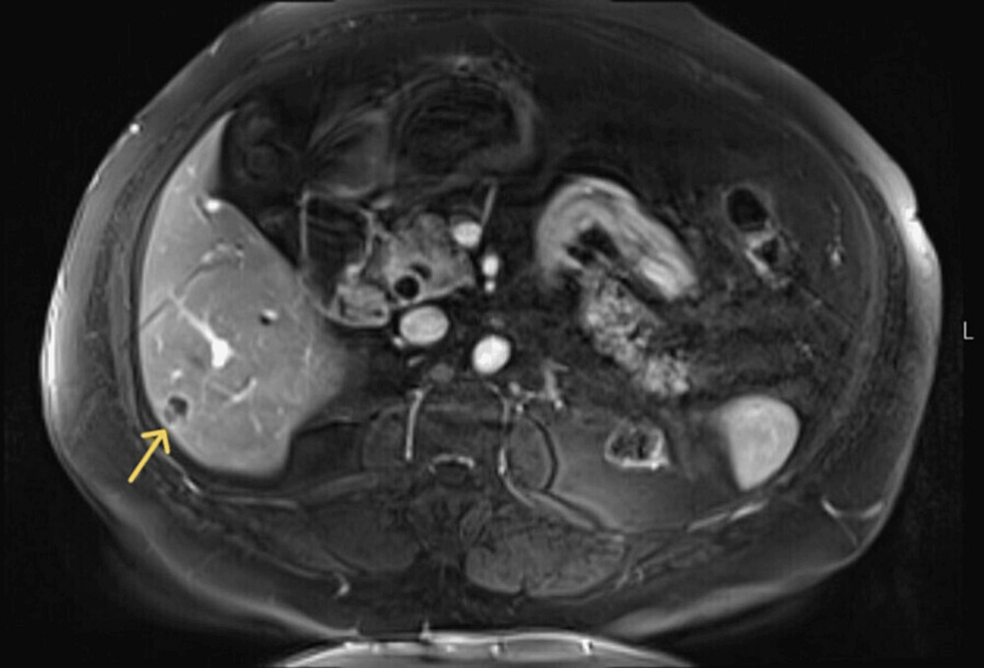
Axial post-contrast fat-suppressed T1-weighted image shows a small segment 6 liver lesion (arrow) with low signal centrally and peripheral enhancement
